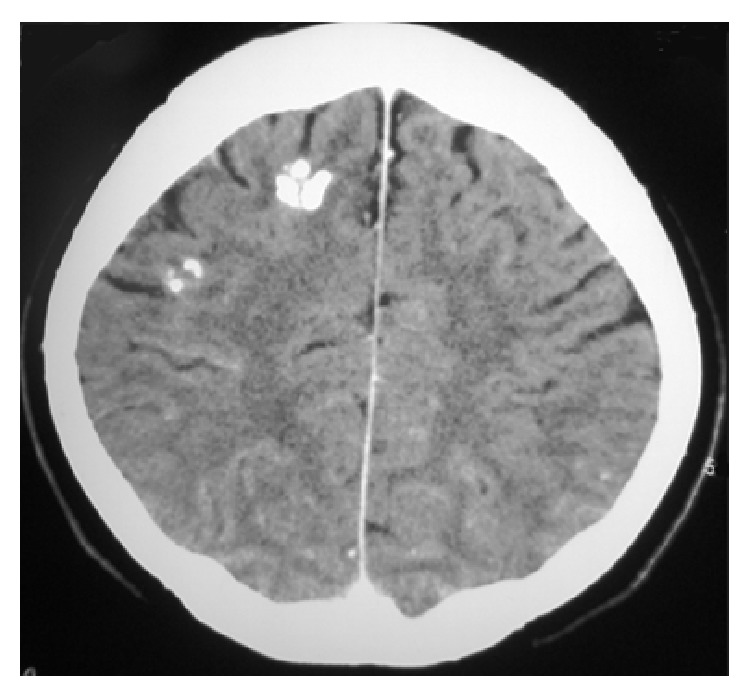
Treated tuberculoma. Axial noncontrast CT image shows two calcified lesions in right frontal lobe without edema or mass effect.
